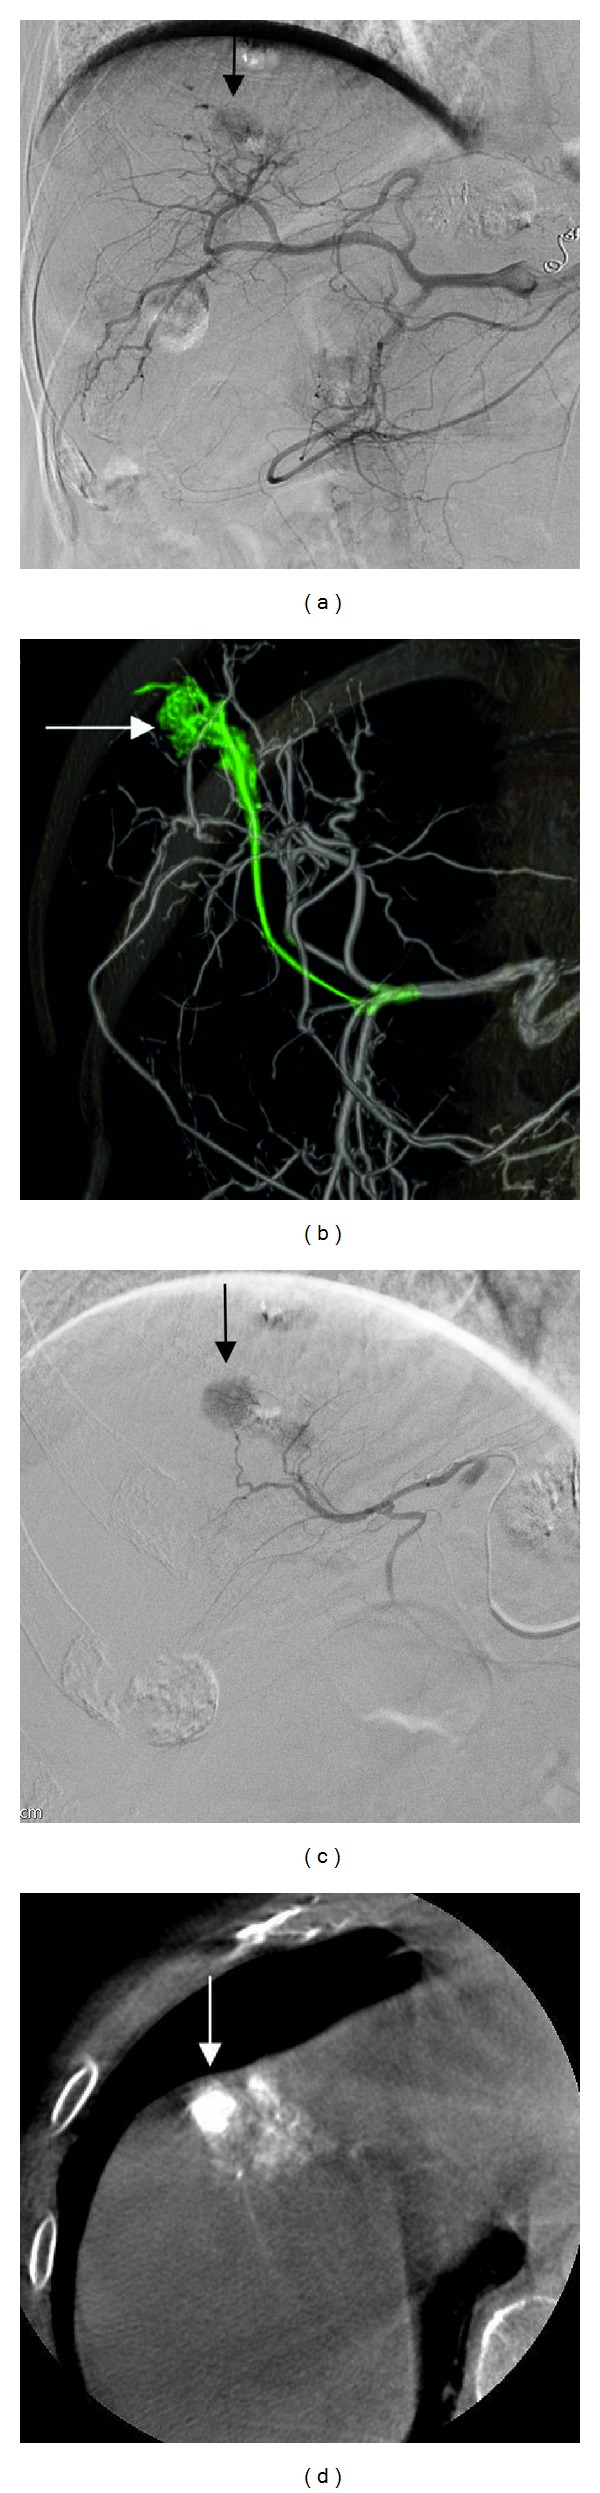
Images from a patient with hepatocellular carcinoma undergoing transarterial chemoembolization with the assistance of automated feeder-vessel detection software. (a) The common hepatic artery angiogram shows the target tumor (arrow) at the right hepatic dome. Identifying the complex arterial vasculature of the tumor feeder is difficult. (b) The volume-rendered C-arm computed tomography (CT) image, showing the extracted tumor feeder by the software as a path from the catheter to the target (arrow) indicated in green. (c) Selective catheterization directly into the suggested feeder artery, based on the software analysis, shows tumor enhancement (arrow). (d) The axial C-arm CT image, obtained during contrast injection from the same feeder artery, confirms target enhancement (arrow) in association with the treatment area.
